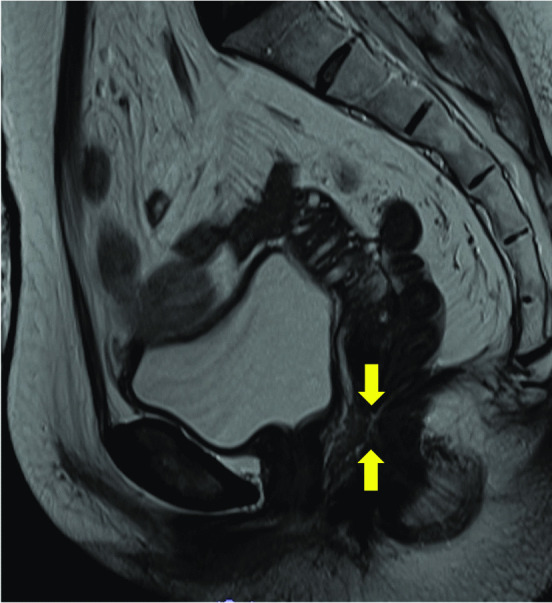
Magnetic resonance imaging shows a fistula between the rectum and vagina (arrows). No pelvic abscesses or recurrent tumors are seen
